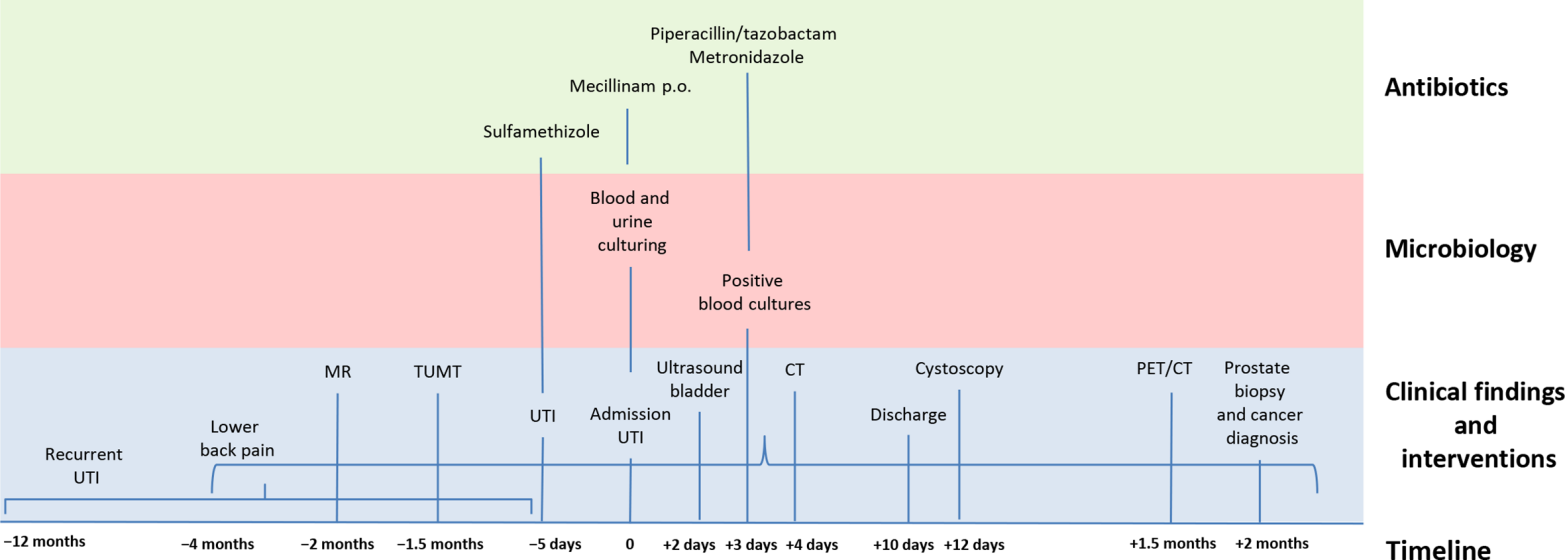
Case timeline. UTI, urinary tract infection; MR, magnetic resonance; TUMT, transurethral microwave therapy, CT, computed tomography; PET/CT, positron emission tomography with CT.
